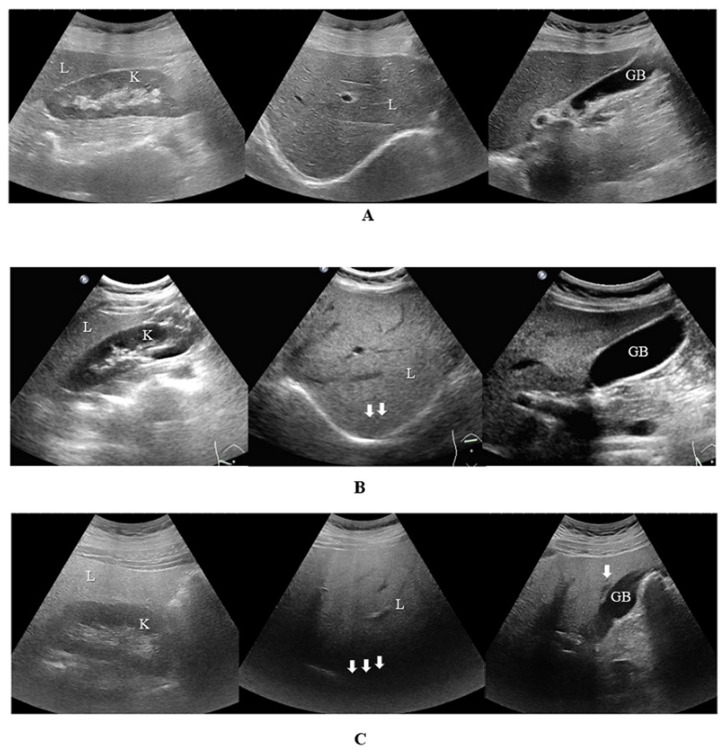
Calculation of US-FLI. Figures ABC show ultrasound images of liver sections in three patients. (A) Comparison of liver and kidney (score 0), US-FLI = 0. (B) Comparison of liver and kidney (score 3), posterior echo attenuation (score 1) (two arrows), blurring of the vessels (score 1), US-FLI = 5. (C) Comparison of liver and kidney (score 3), posterior echo attenuation (score 1), blurring of the vessels (score 1), unclear display of gallbladder wall (score 1), difficulty in displaying diaphragm (score 1) (three arrows), difficult visualization of the areas of focal sparing (score 1) (one arrow), US-FLI = 8. Abbreviations: L, liver; K, kidney; GB, gallbladder.
